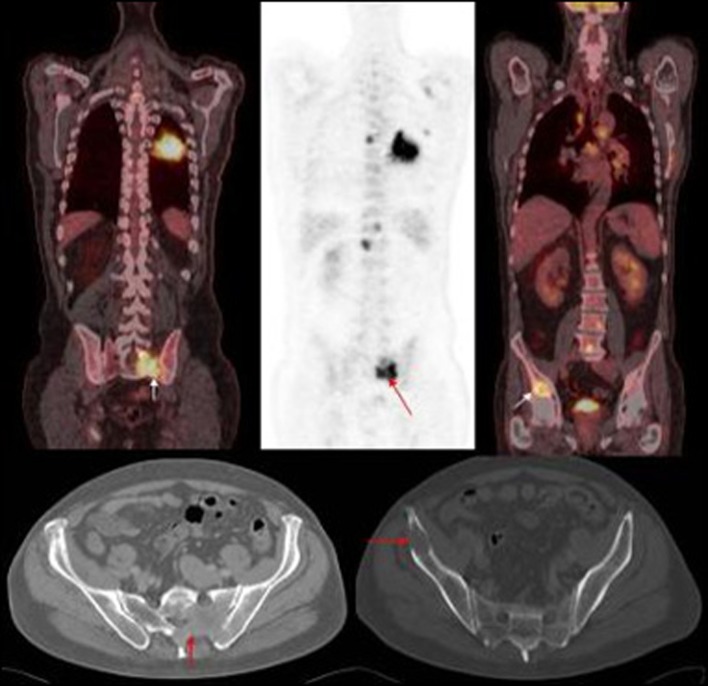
PET images of primary left lung cancer metastatic deposits in the bony pelvis and lumbar vertebrae with corresponding axial CT images of the pelvis.
